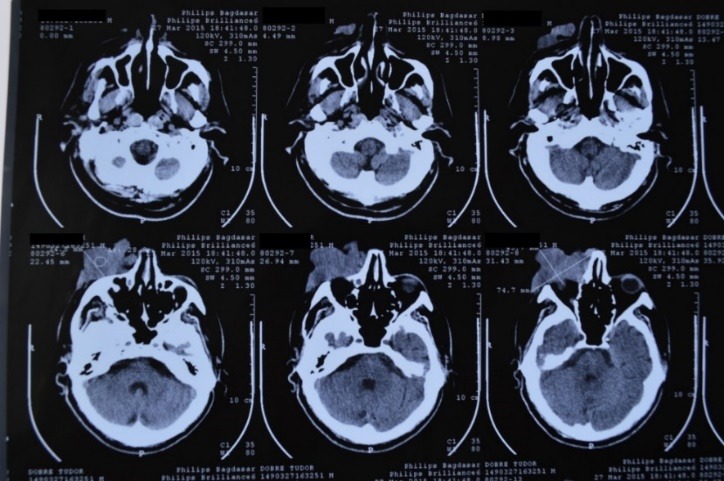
Anatomic relations of the tumor with the ophthalmic, nasal, maxillary, and zygomatic regions (computed tomography examination)
